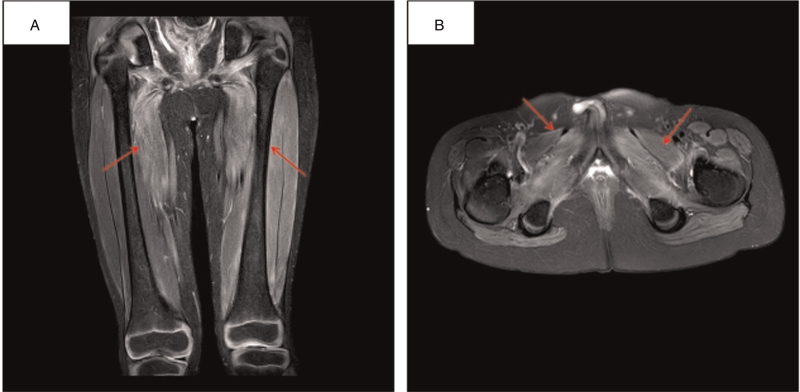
MRI imaging. Fat-suppressed T2-weighed imaging showed high intensity in the left thigh and pelvic muscles (a, b).
